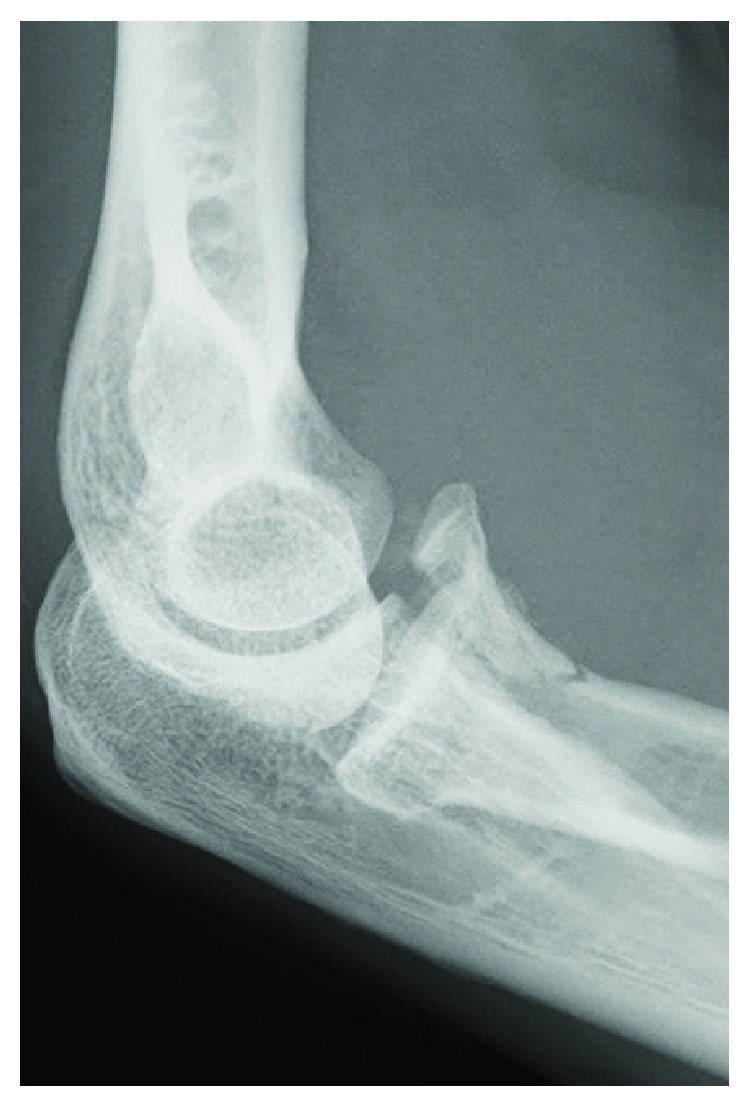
Case 3: radiograph demonstrates a type 2 coronoid fracture.
